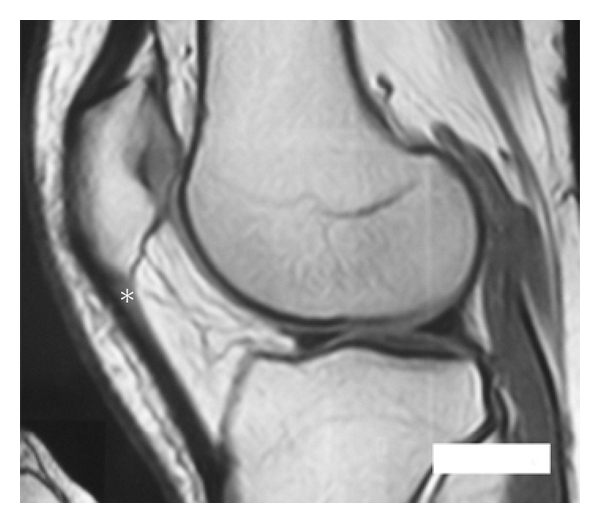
Preoperative magnetic resonance imaging showing hypodens area in the proximal aspect of the patellar tendon (*).
